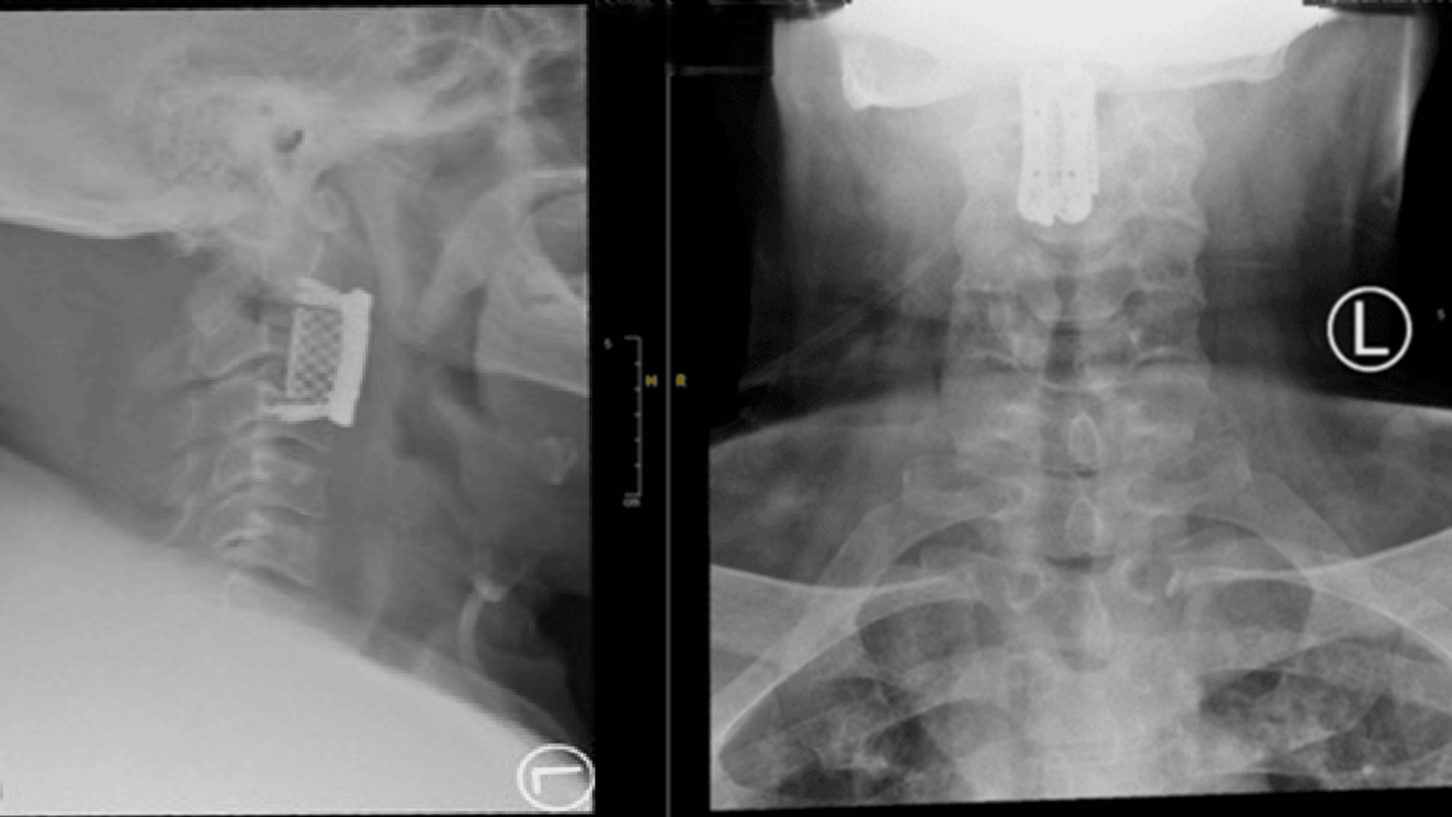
Post-operative radiograph showed a satisfactory position of the cage.
